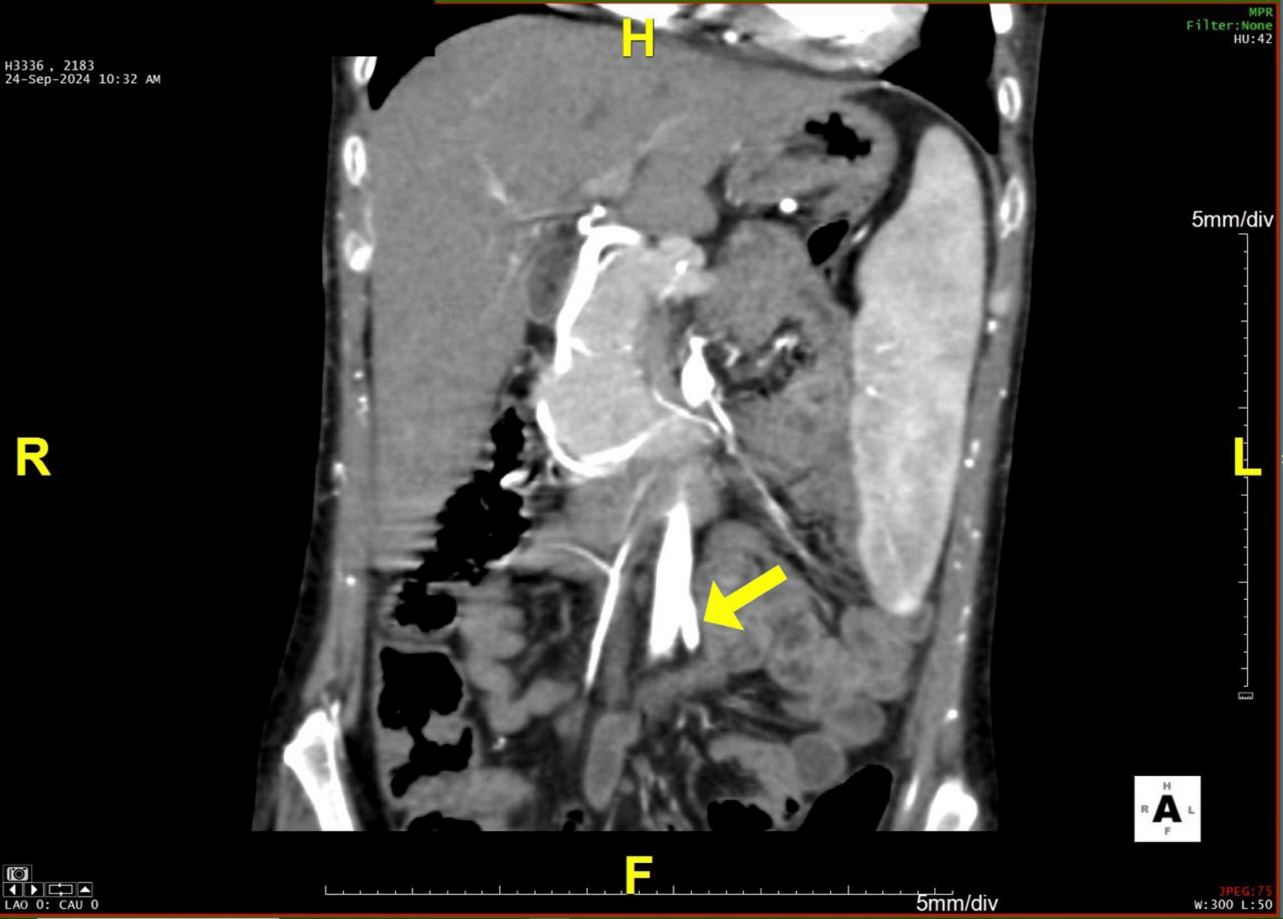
A coronal section of the CT angiogram reveals sparing of the inferior mesenteric artery CT: computed tomography
